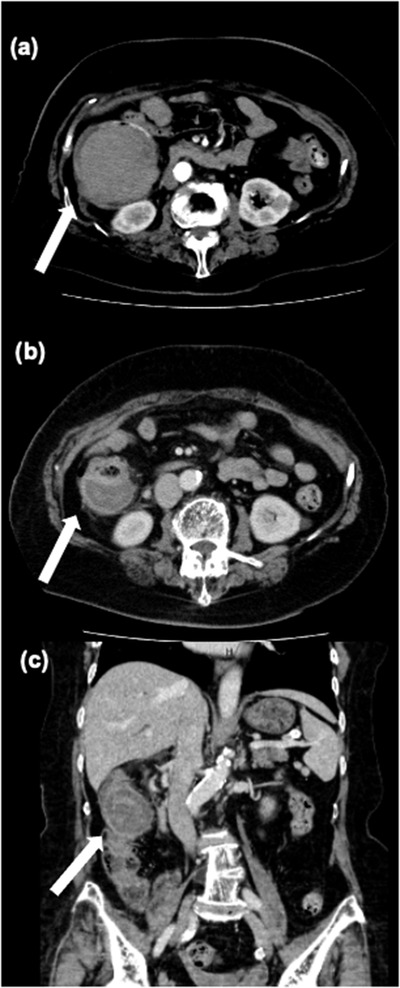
Contrast‐enhanced computed tomography 6 and 16 days after cold snare polypectomy (CSP): (a) hematoma (white arrow) 6 days after CSP, unchanged; (b) hematoma (white arrow) was decreased to about 45 mm in 16 days after CSP; and (c) coronal view of the hematoma (white arrow) 16 days after CSP
